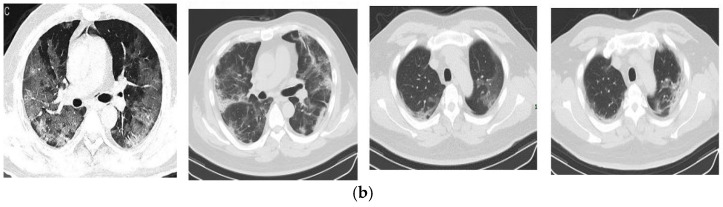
Some samples of COVID-CT-349 dataset. (a) Non-COVID-19 cases, (b) COVID-19 patients.
